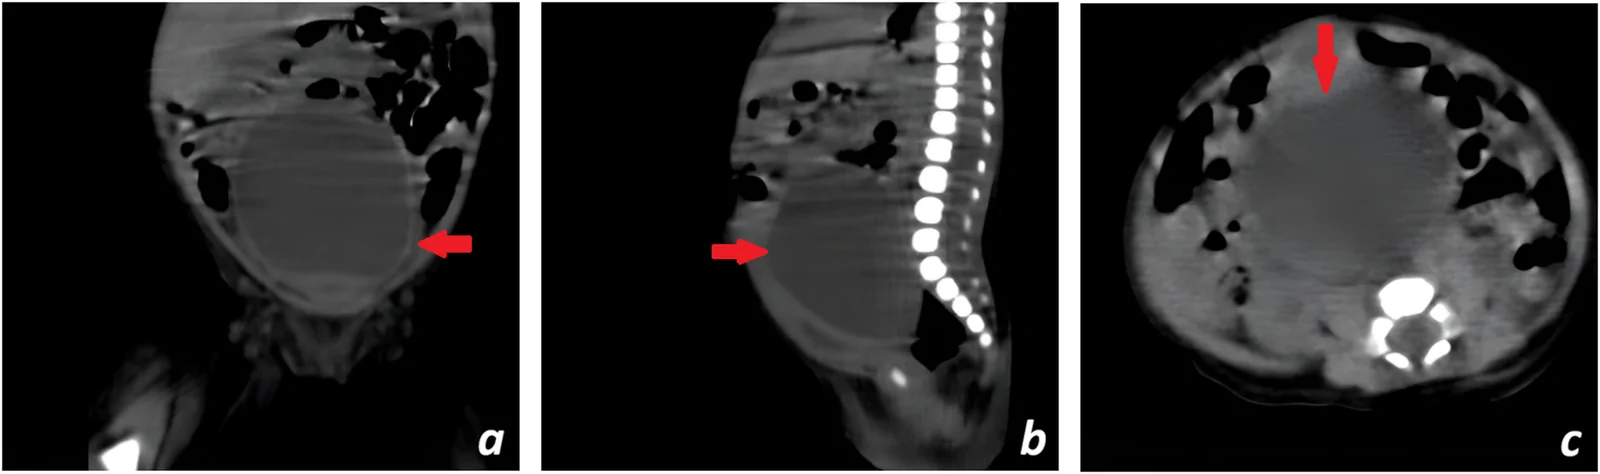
Abdominopelvic computed tomography (CT) showing a cystic lesion measuring 57 × 43 × 64 mm, which is observed superior to the urinary bladder and extends into the pelvis, consistent with hydrometrocolpos (a: coronal, b: sagittal, c: axial views)
